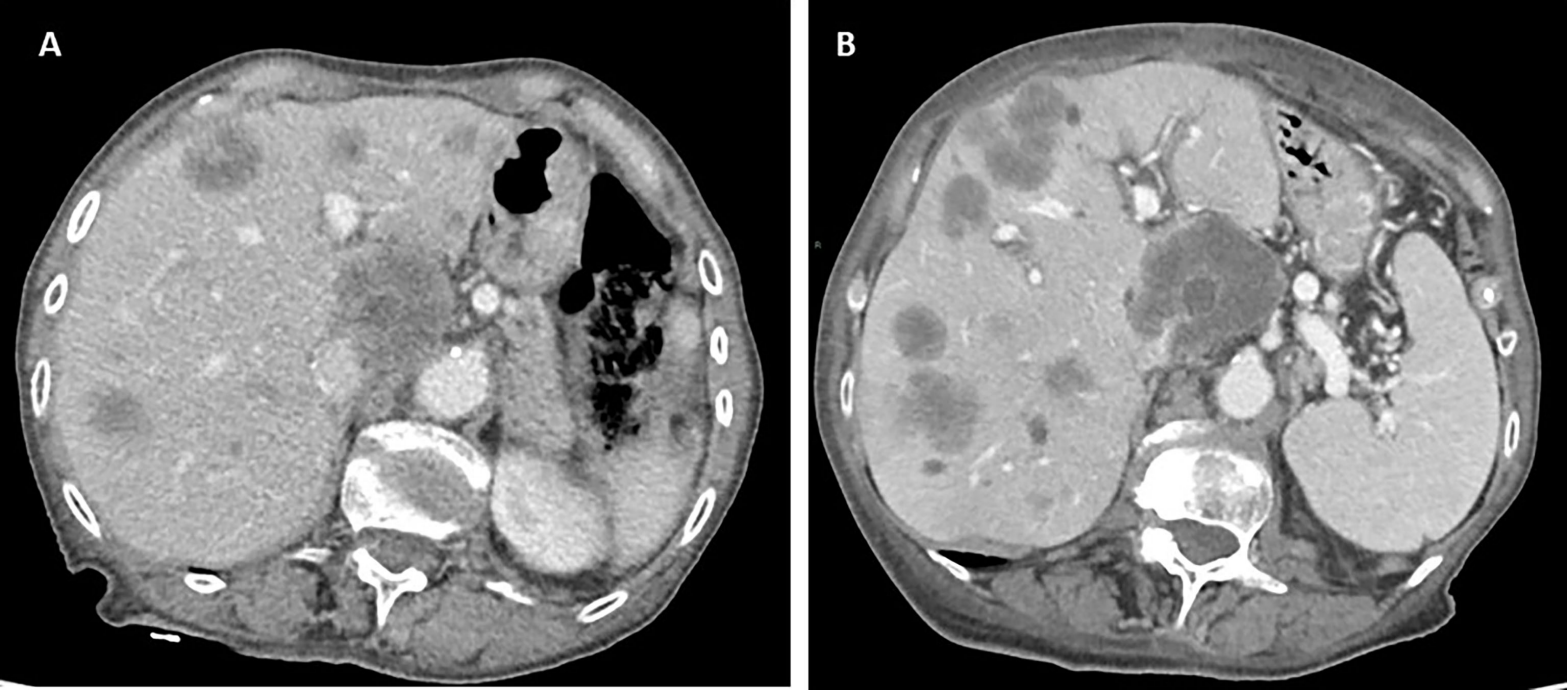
Computed tomography (CT) scan evaluation in patient 5 before (A) and after (B) 3 months of systemic treatment, showing rapid disease progression to the liver.
